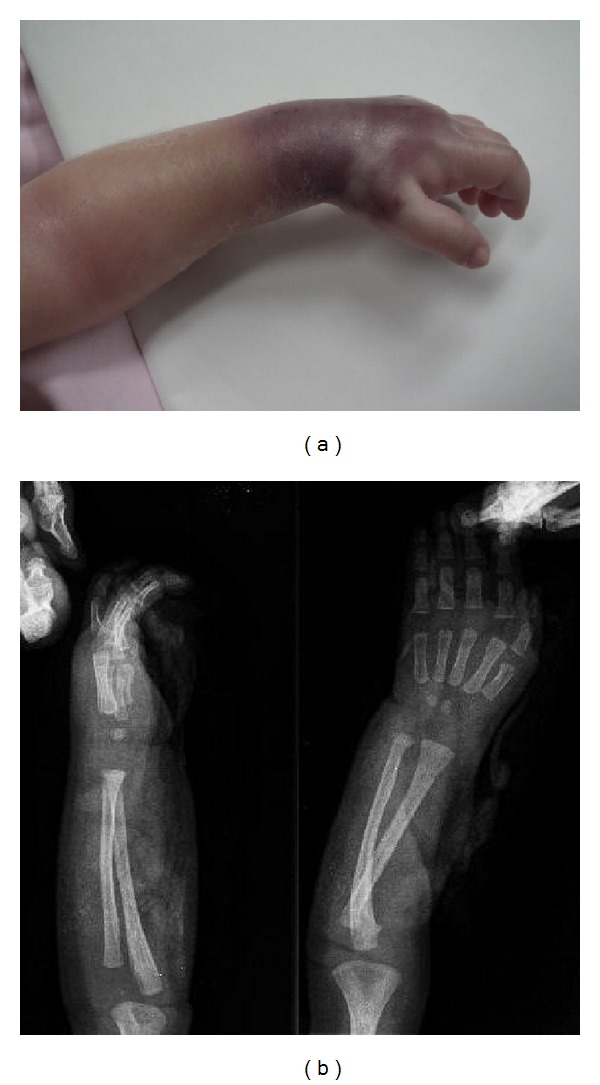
(a) Image showing the left hand and the forearm of the patient. Remarkable edema, swelling extending through the forearm can be observed. (b) X-ray of the patient shows a cloudy image indicating a considerable accumulation of contrast agent of both the forearm and the hand.
